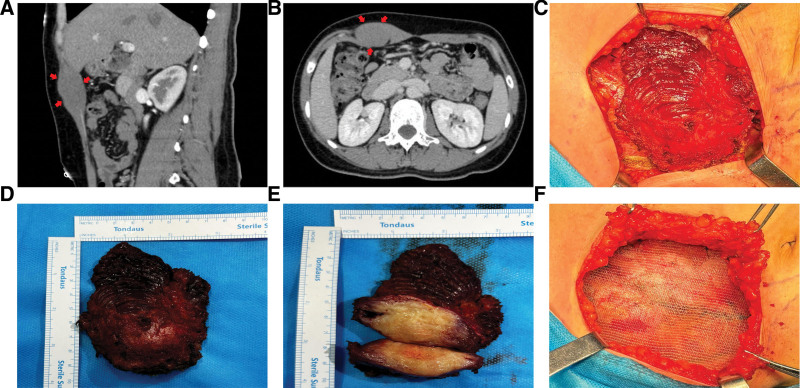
A 32-year-old female presented with DT of the right rectus abdominis, showing preoperative CT scans (A, B), surgical resection of the tumor (C–E), and patch reinforcement for repairing the abdominal wall (F). DT = desmoid tumor.
